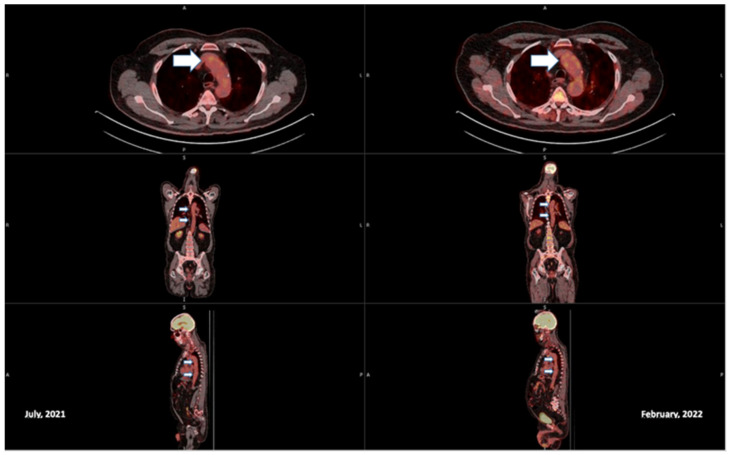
Baseline and fused PET/CT transaxial, coronal and sagittal images; arrows indicate aortic uptake.
